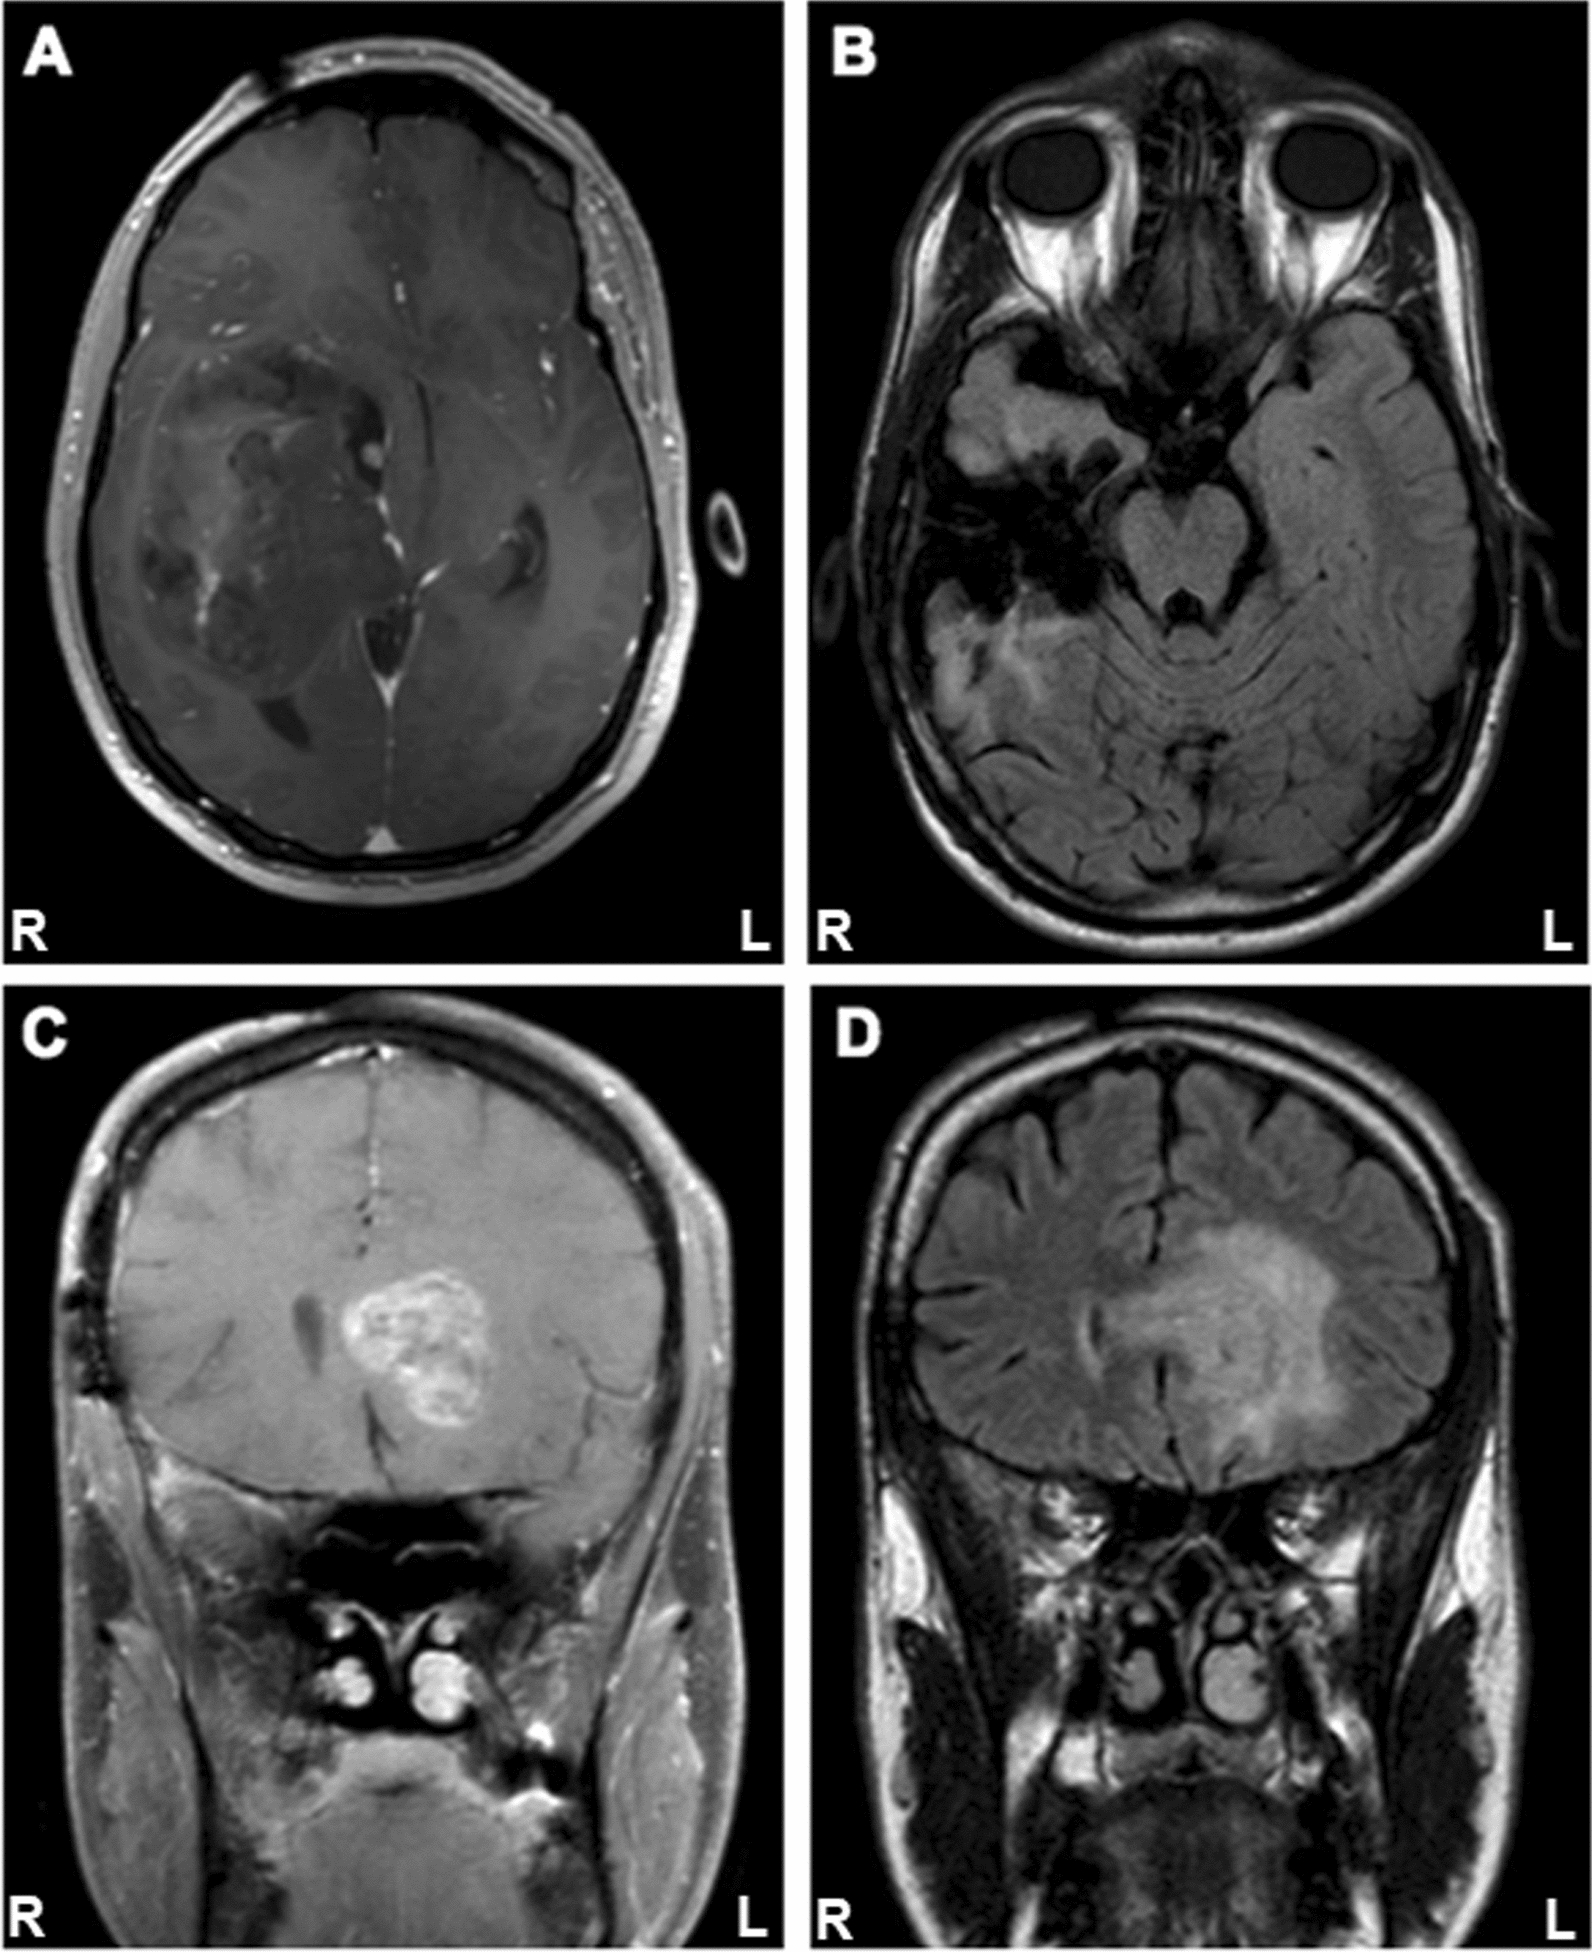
MRI panels demonstrating A gadolinium contrast enhancing T1 of initial tumor centered in right temporal/parietal lobe with right-to-left shift (transverse section), B the resection cavity immediately following surgery (transverse section), C gadolinium contrast enhancing T1 of recurrent tumor with migration to left hemisphere (coronal section), and D T2 FLAIR imaging of recurrent tumor with migration to left hemisphere (coronal section)
